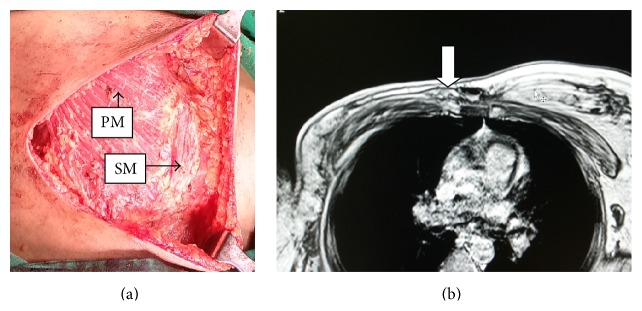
(a) Intraoperative photograph showing a thin fleshy ribbonlike muscle of size approximately 10 cm × 3 cm present in the right parasternal region perpendicular to fibres of the pectoralis major muscle. PM: pectoralis major, SM: sternalis muscle. (b) MRI of anterior chest wall (post-MRM) showing a unilateral sternalis muscle (white arrow) in transverse section.
